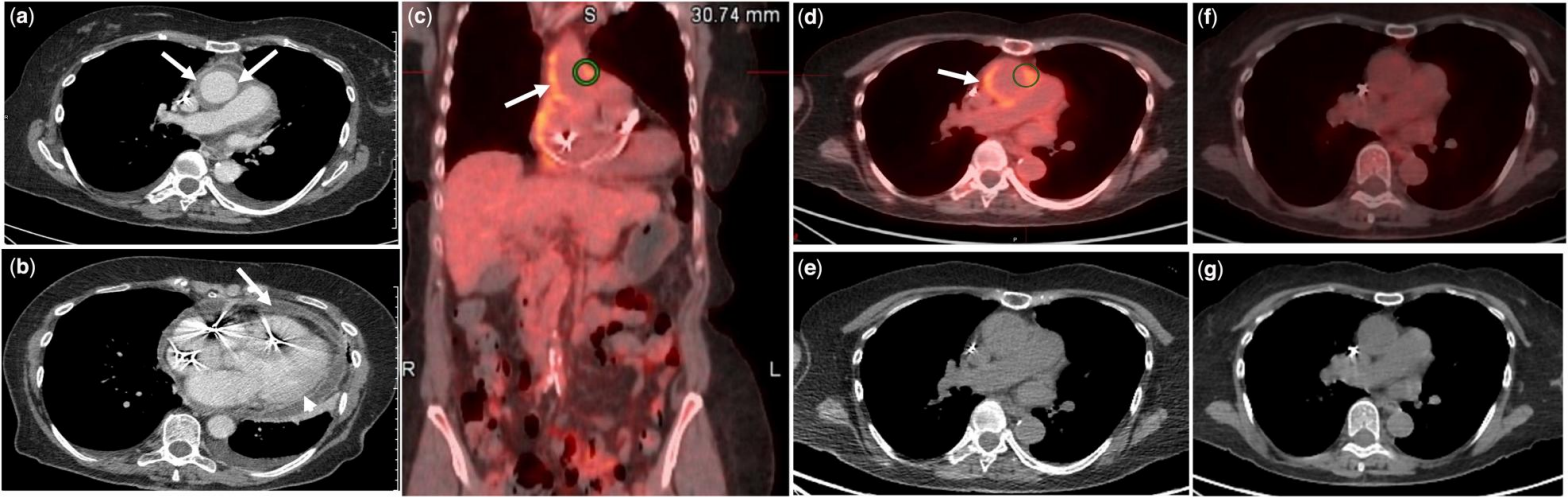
Whole-body FDG PET/CT showing pericarditis and vasculitis at baseline and resolution after 4 months of follow-up. (a) Axial enhanced chest CT scan, which revealed circumferential aortic wall thickening with enhancement and mild fat stranding (arrows). (b) Axial enhanced chest CT scan, which revealed pericardial wall thickening with mild enhancement (arrowhead), which indicates pericarditis and pericardial effusion (arrow). (c) Fused coronal FDG PET/CT scan, demonstrated an increased FDG uptake over the wall of the ascending aorta and pericardial wall (arrow). (d) Fused axial FDG PET/CT scan demonstrated an increased FDG uptake over the wall of the ascending (arrow). (e) Axial non-enhanced chest CT scan, which revealed circumferential aortic wall thickening with mild fat stranding (arrow). (f) Four-month follow-up. Fused axial FDG PET/CT scan demonstrated normal FDG distribution with no abnormal uptake. (g) Four-month follow-up axial non enhanced CT scan shows no abnormality
